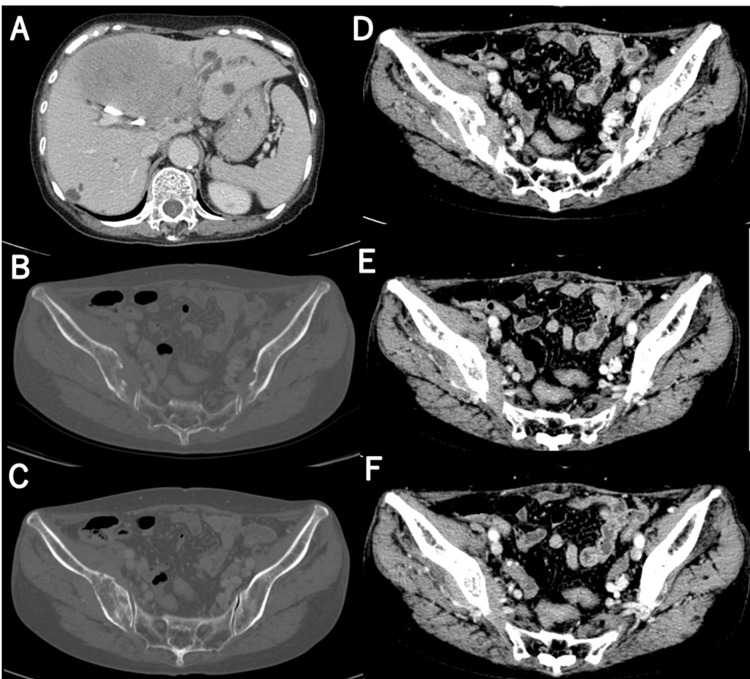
CT scan on admission. (A) CT scan shows the multiple mass in the liver and a biliary stent; (B) it also revealed the osteolytic change; (C) a pathological fracture of the right iliac bone near the sacroiliac joint; and (D-F) a mass of soft tissue density around it and extended continuously to the right ischial foramen.
